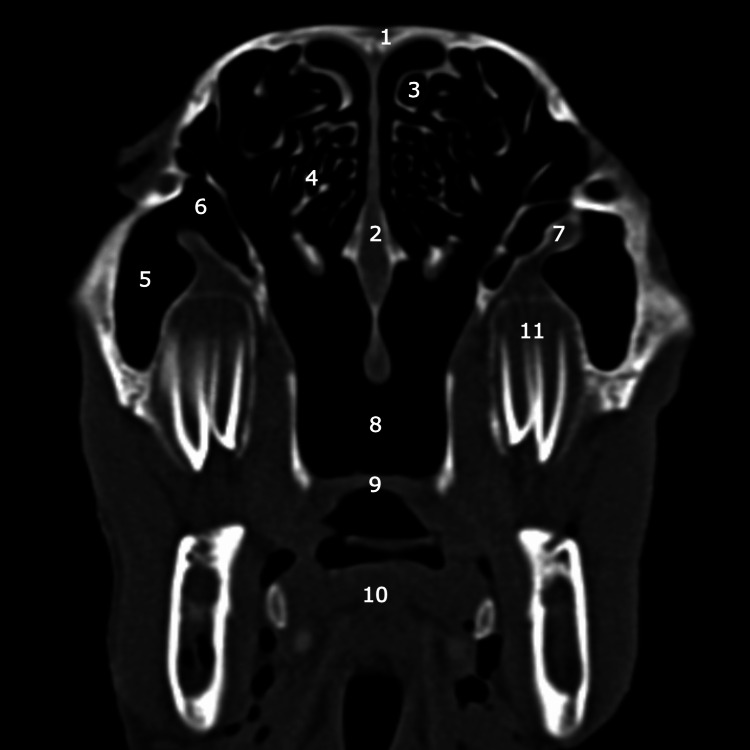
Coronal CT scan at the level of the third molar tooth 1: frontal bone; 2: nasal septum;3: pneumatized superior turbinate; 4: ethmoidal labyrinth;5: lateral chamber of maxillar sinus; 6: medial chamber of maxillar sinus; 7: infra: orbital canal; 8: posterior septal defect; 9: hard palate; 10:  body of tongue; 11: third molar tooth.
